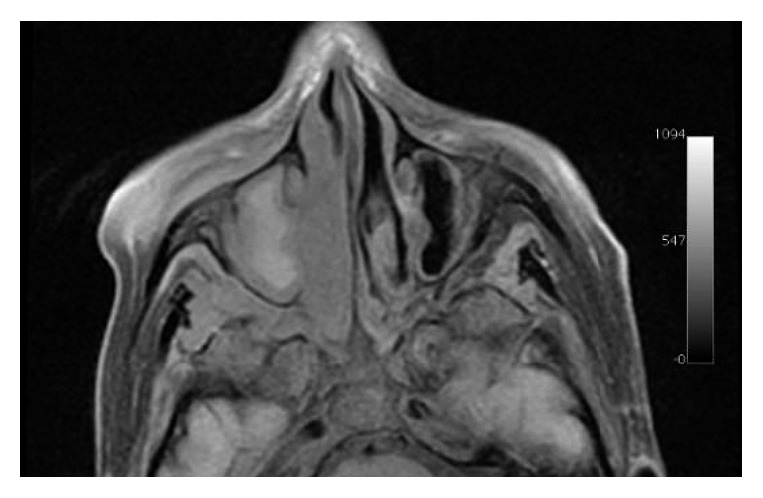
T1-weighted MRI with fat suppression demonstrates the lesion extending to the pterigomaxillary fissure.
